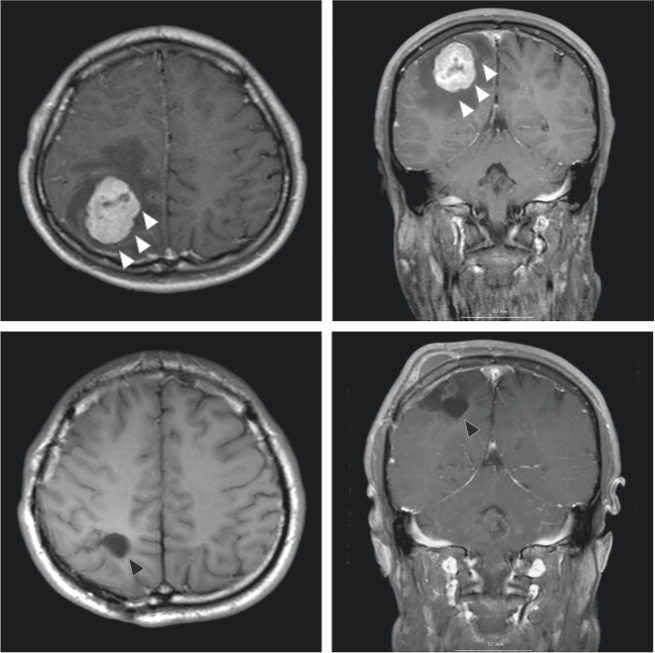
Magnetic resonance imaging of the brain metastasis. Above, representative axial and coronal T1-weighed gadolinium-enhanced Magnetic resonance imaging (MRI) slices of the brain at the time of seizure. White arrow: brain tumor. The images reveal a 45-mm mass on the right side of the brain. Below, axial and coronal T1-weighed gadolinium-enhanced MRI slices, taken 6 months post-operation. Black arrow: post-treatment change after operation.
